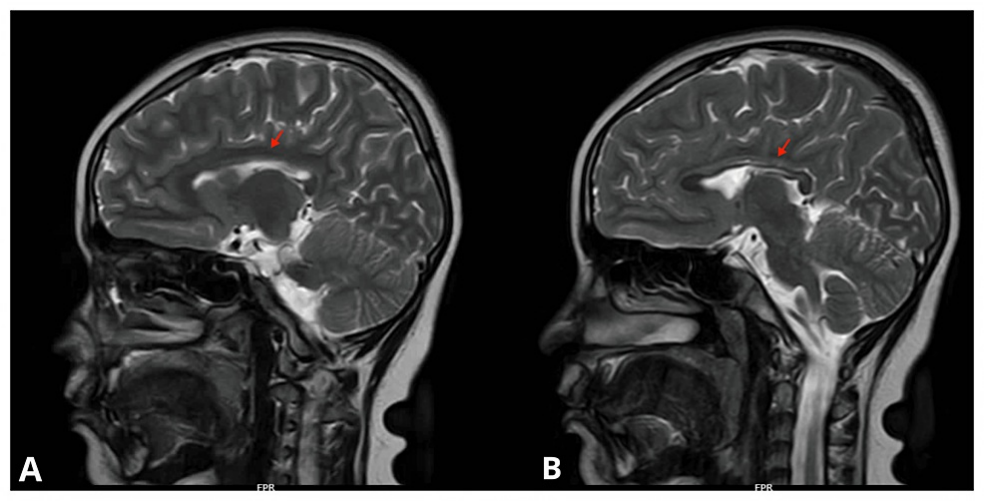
MRI of the patient showing thinned-out posterior corpus callosum (red arrow). A & B: sagittal section, sequence 4, T2-weighted imaging.
